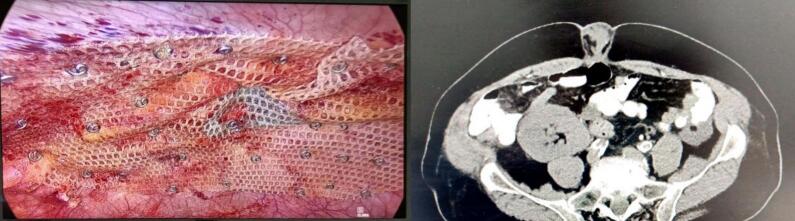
CT scan, axial plane, where the strangulated paraumbilical hernia appears, and the endoscopic repair site.
